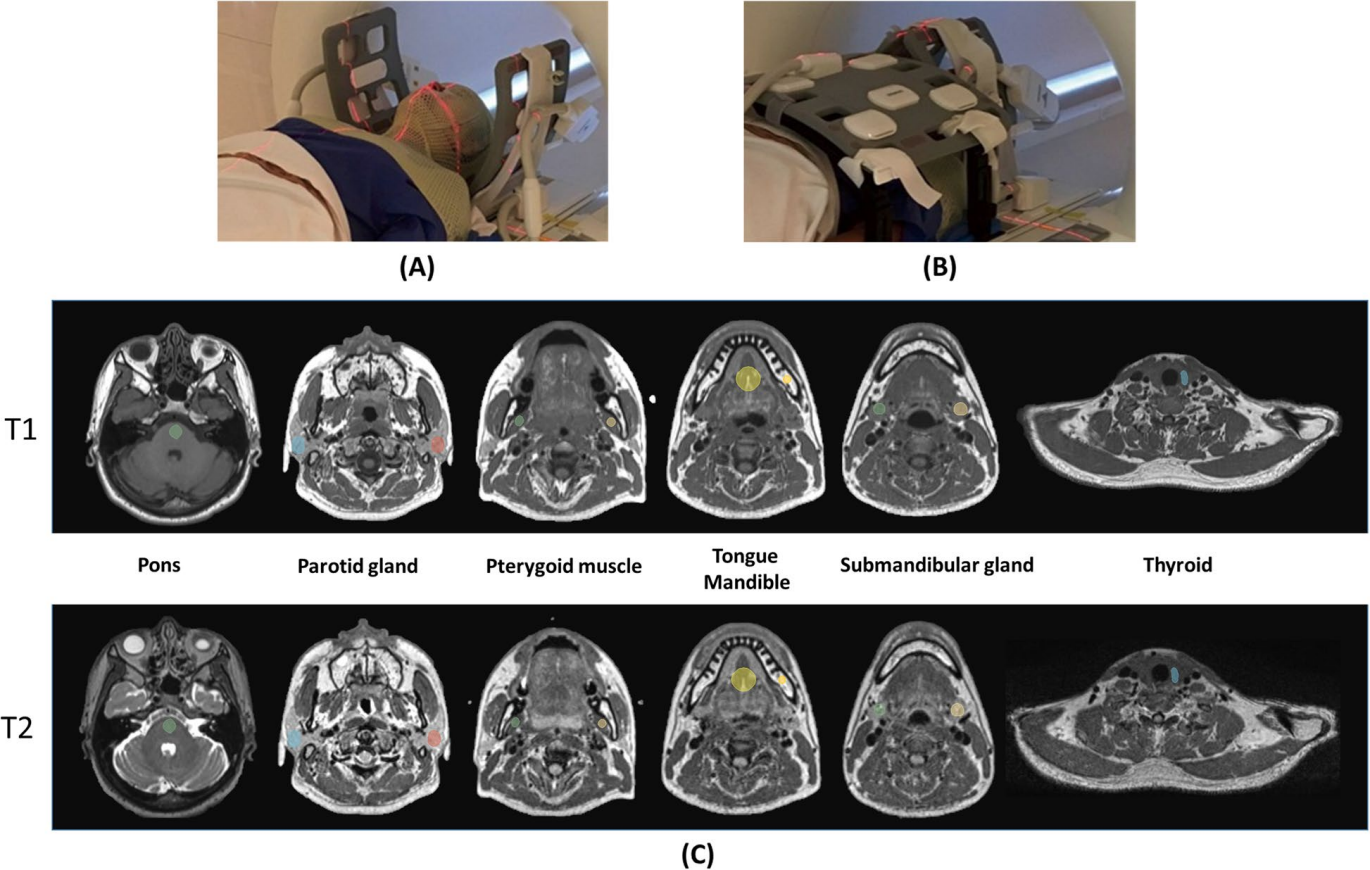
Subject positional verification setup and the acquired 3D TSE MR images. (a) A subject immobilized using thermoplastic mask aligned with the external laser system; (b) a subject setup and the coil setting on the MR-sim; (c) all ten VOIs on MRI image
